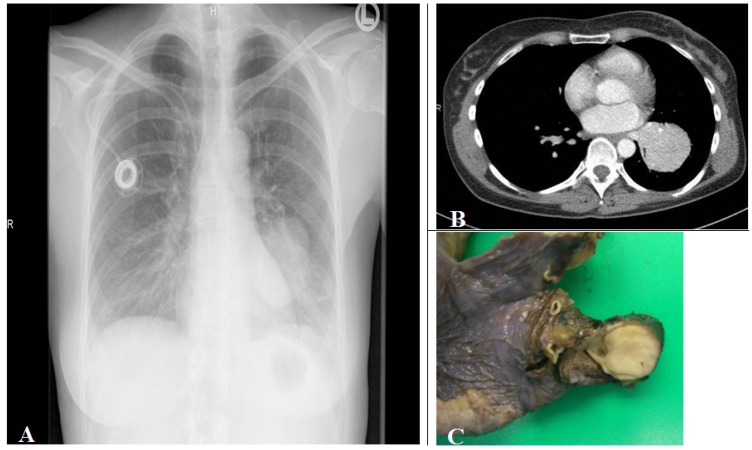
(A) Corresponding chest radiograph and (B) thorax CT scan (parenchymal window) of a patient with a tissue density/mass (left lower lobe compression) induced by an intrabronchial localized carcinoid tumor demonstrated in the lobe resection tissue (C). The tumor is seen as white to yellow tan intrabronchial tissue mass.
